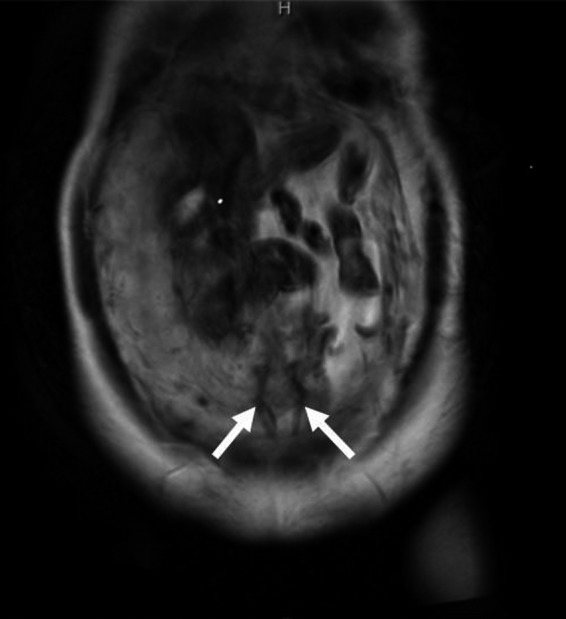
T2‐weighted MRI confirmed that the cord vessels were running on the anterior lower uterine segment. The white arrows indicate the cord vessels
